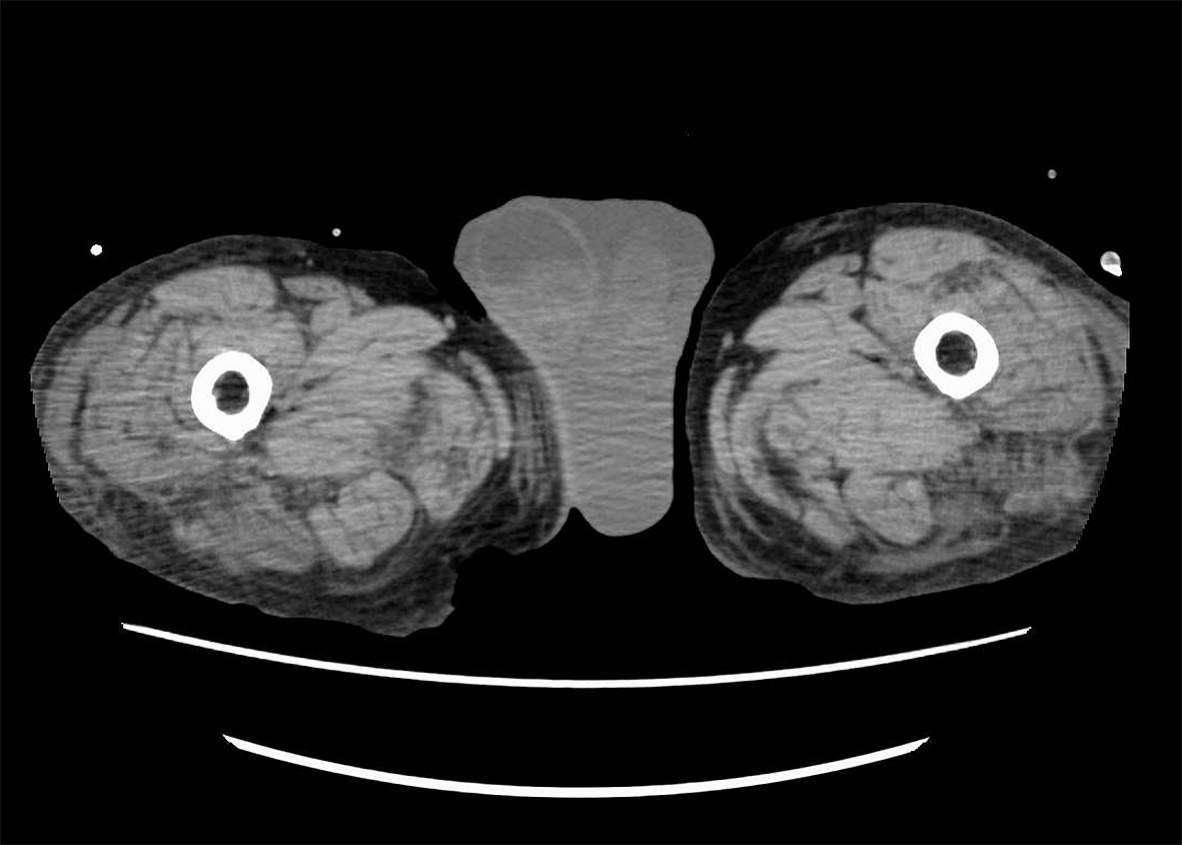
Computed tomography showed scrotum with fluid collection.
